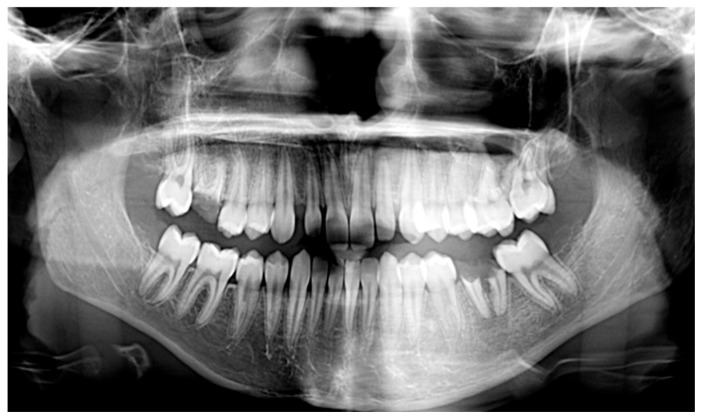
Patient n.3: pre-treatment panoramic radiograph shows rarefying osteitis associated with destructive caries of 3.6.
